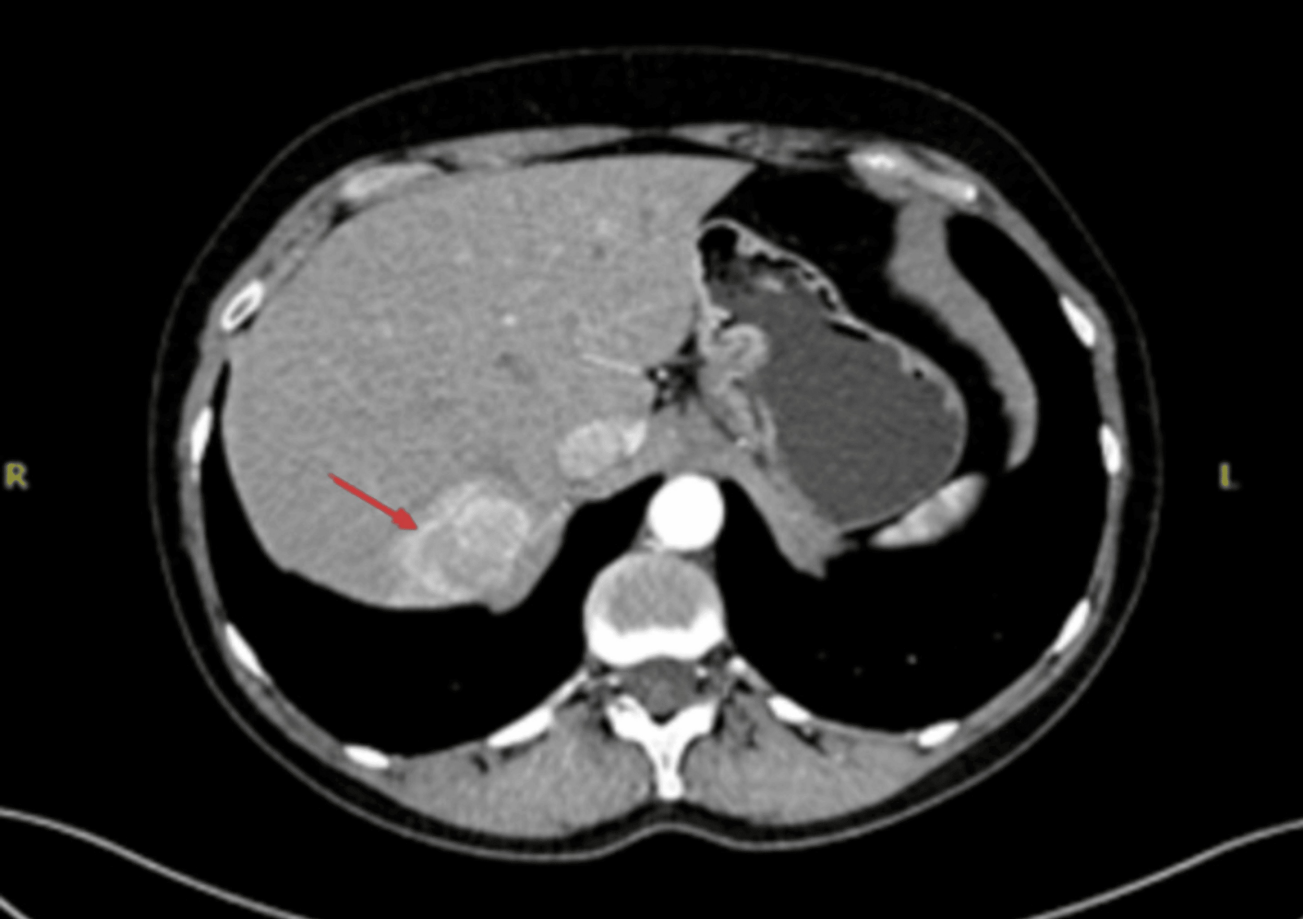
Initial CT of the abdomen reported multiple liver haemangiomas (red arrow).
